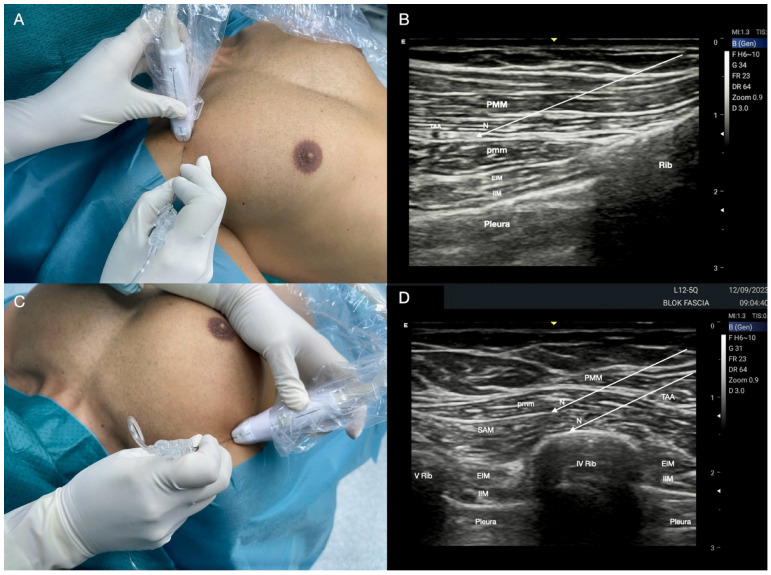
Interpectoral plane (IPP) and Pectoserratus Plane (PSP) blocks. (A) IPP block, patient and probe position. The patient is placed in the supine position with the head rotated in the opposite direction from the injection site, exposing the deltoid-pectoral groove. A linear high-frequency probe is positioned in the deltoid-pectoral groove. The needle is inserted with the in-plane approach in a medial-to-lateral direction or in a lateral-to-medial direction. (B) IPP block, ultrasound landmarks and local anaesthetic injection. From top to bottom: pectoralis major muscle, pectoralis minor muscle, pectoral branch of the thoracoacromial artery, rib, external and internal intercostal muscles, pleura. The local anaesthetic is injected between the pectoralis major and minor muscles following the pectoral branch of the thoracoacromial artery. (C) PSP block, patient and probe position. The probe slides towards the axilla at the level of the fourth rib. The needle is inserted with the in-plane approach in a medial-to-lateral direction. (D) PSP block, ultrasound landmarks and local anaesthetic injection. Local anaesthetic is injected between the pectoralis minor and the serratus anterior muscles or between the serratus anterior muscle and the intercostal muscle at the level of the fourth rib. PMM: pectoralis major muscle; pmm: pectoralis minor muscle; SAM: serratus anterior muscle; IIM: internal intercostal muscle; EIM: external intercostal muscles; TAA: pectoral branch of the thoraco-acromial artery; Rib: rib; Pleura: pleura; N: needle.
